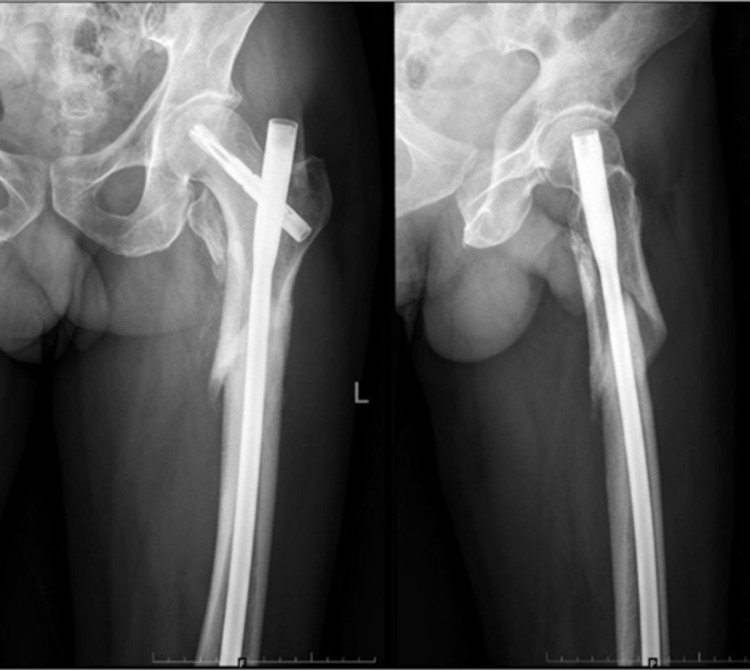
Six weeks follow-up left hip - AP (L) and lateral (R) radiographs AP: anteroposterior
